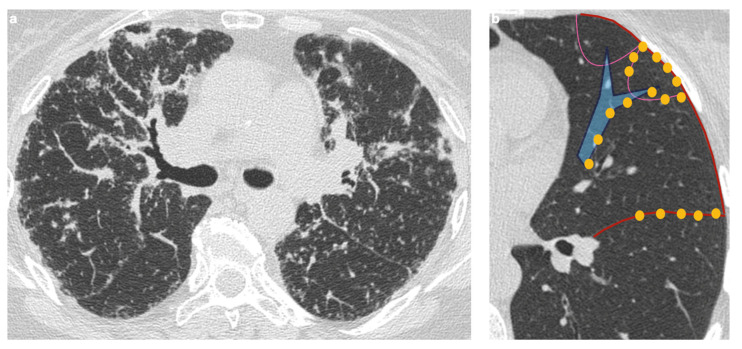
(a) High-resolution CT scan in the axial plane shows the presence of diffuse micronodules with a peri lymphatic distribution. (b) Those well-defined micronodules (yellow points) are typically seen along bronchi (blue lines), interlobular septa (pink lines), fissures and pleura (red line).
